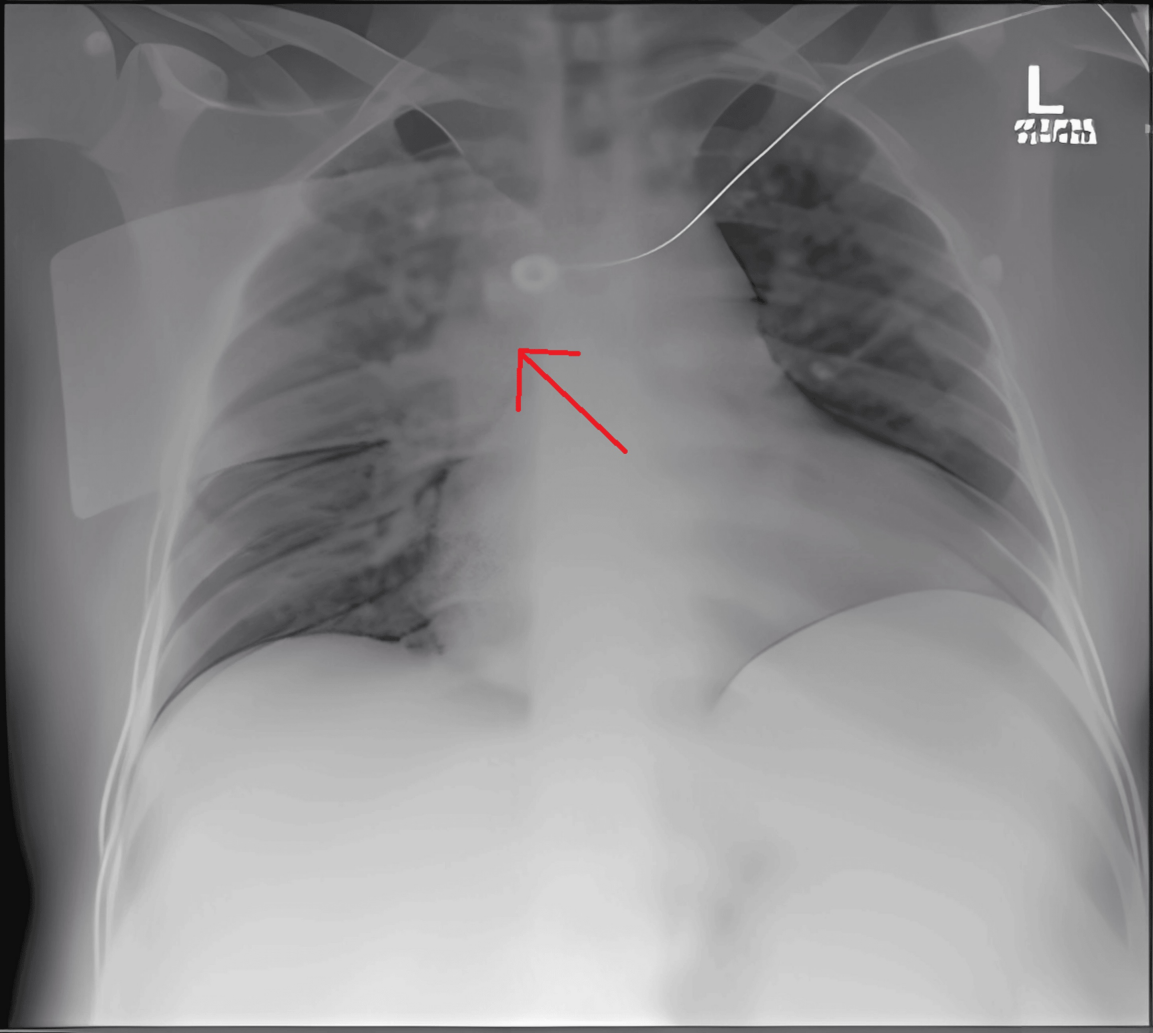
Chest X‐ray demonstrating the temporary pacing lead within the right ventricle Chest X-ray demonstrating the faint temporary pacing lead (arrow) within the right ventricle. The lead can be seen tracing from the left subclavian area across the midline into the right ventricle.
